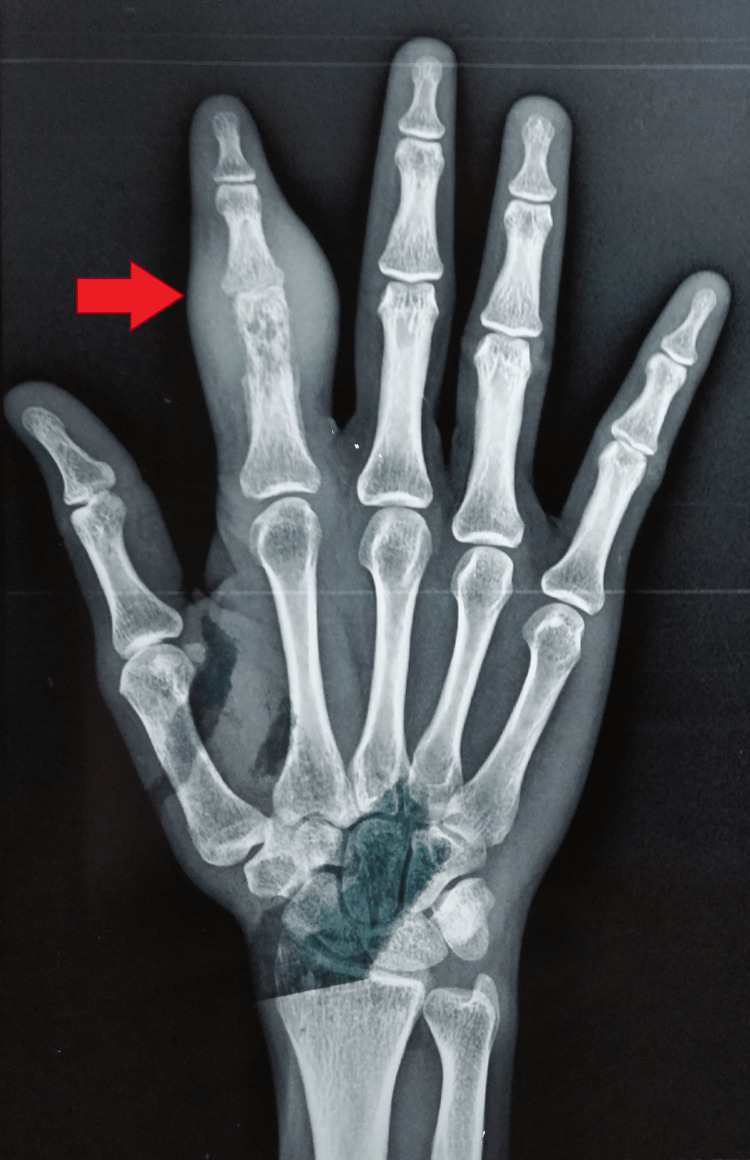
Radiograph of the right hand (P-A view) showing soft tissue swelling with cortical erosion in the distal part of the proximal phalanx P-A: postero-anterior
